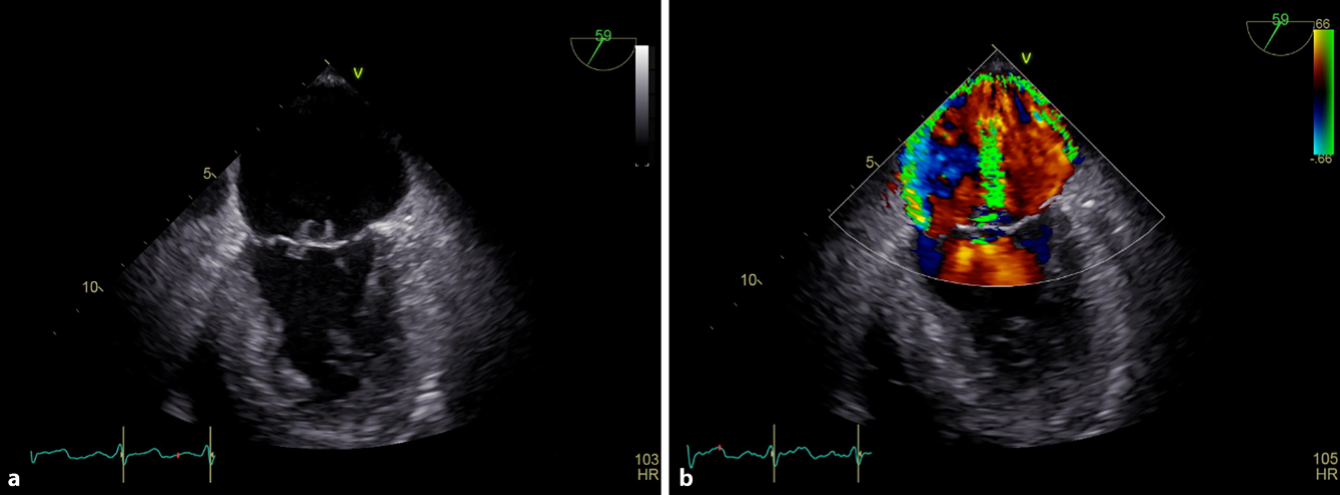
Two-dimensional mid-esophageal commissural view of the mitral valve (a) and its concomitant color doppler (b)
